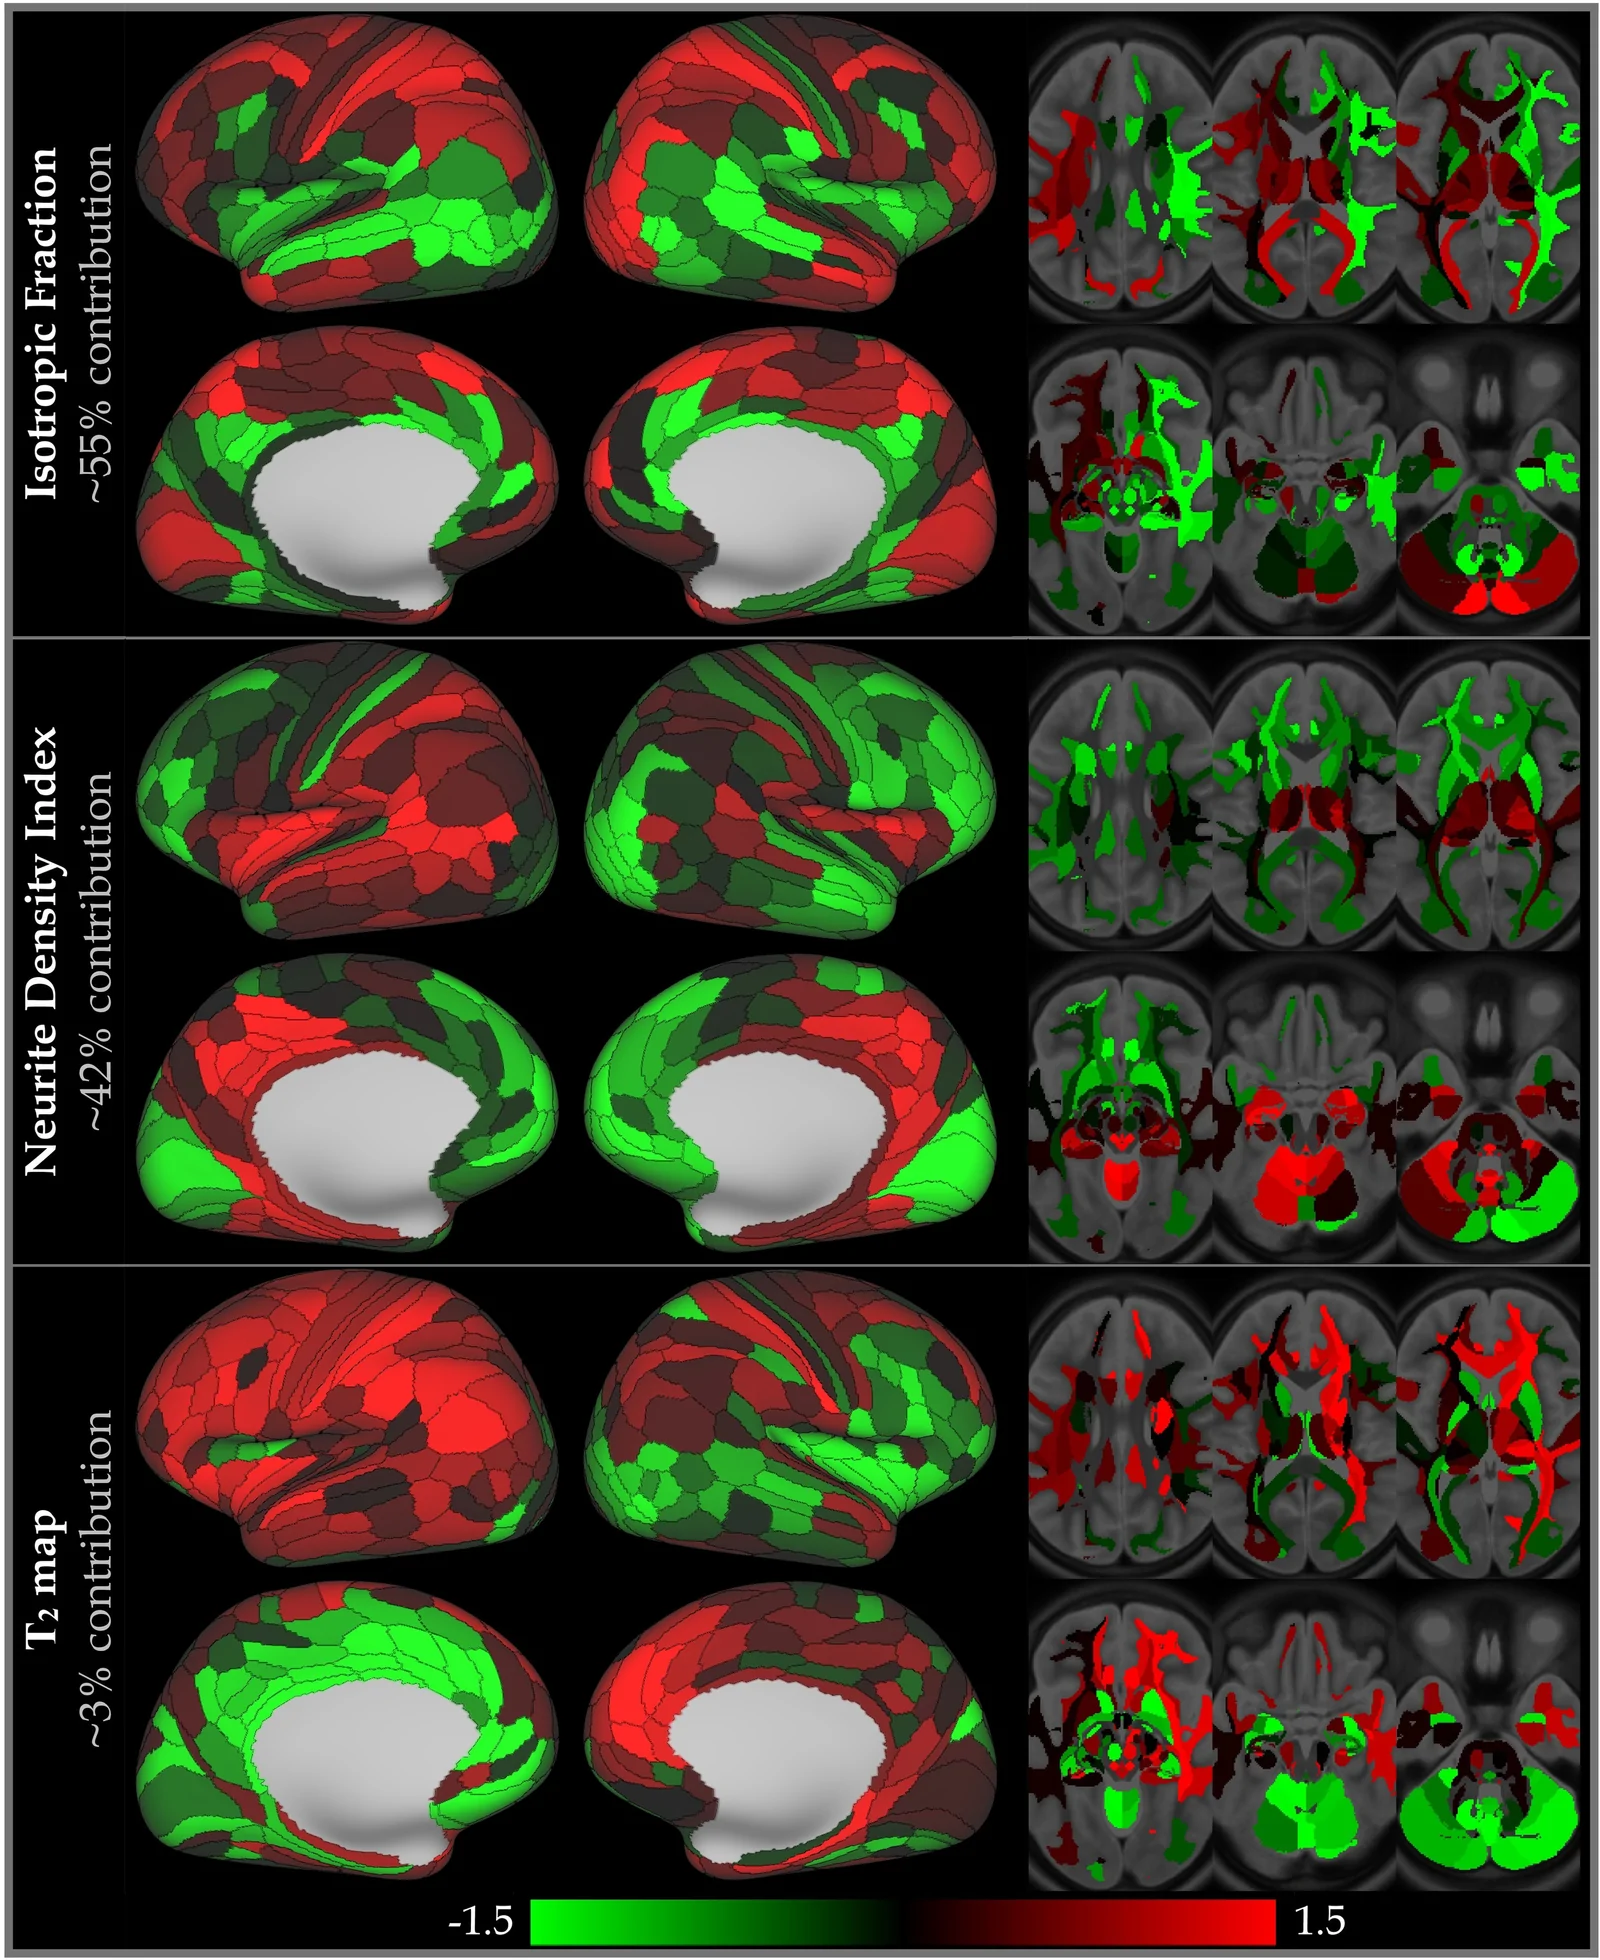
Anatomical mapping of MRI contributions to the brain–cognition axis. Parcel-wise maps show the projection of the discriminant weights back to anatomy. Left panels show lateral and medial cortical surfaces; right panels show subcortical axial slices in Montreal Neurological Space [*z* = 28, 16, 4, −8, −20, −32]. Images follow neurological convention (right = right). Colours encode within-metric normalized parcel weights (scale bar −1.5 to +1.5), with red indicating more positive (Long COVID-like) and green more negative (closer to healthy controls) association with the brain–cognition axis. NODDI isotropic fraction components jointly carried ∼55% of the MRI block’s absolute discriminant weight (‖*w*‖_1_), followed by NDI (∼42%); the T_2_ map component contributed only a small share (the values indicate each metric’s share of the MRI block contribution to the brain–cognition axis, not the total including cognition). Prominent spatial patterns involve fronto-parietal/dorsal-attention and cingulo-opercular–salience networks with thalamo-striatal and cerebellar circuits. See Supplementary Table 4 for a full list.
